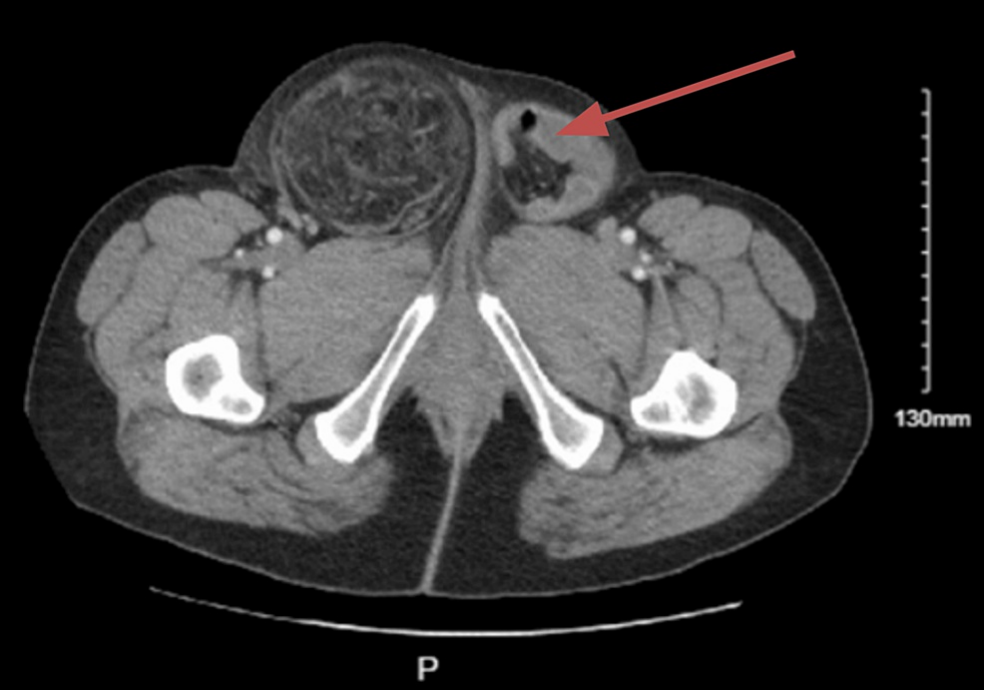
Preoperative CT scan. Bilateral inguinal hernias noted, left with bowel. Bilateral hydroceles are also present. The red arrow points to the testis visualized only after postoperative reinterpretation.
